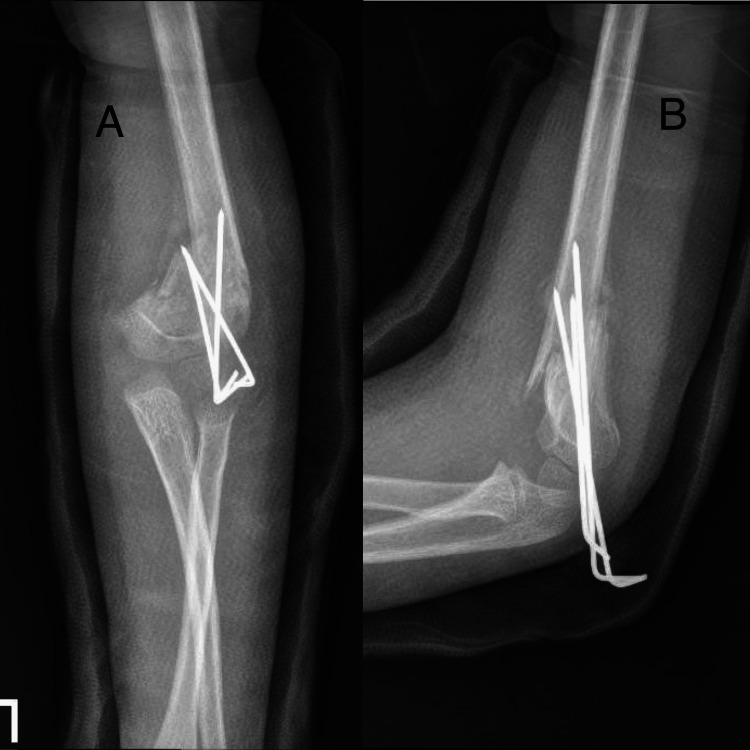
X-ray immediately before revision surgery (day 15 post initial CRPP) (A) anteroposterior view; (B) lateral view CRPP: closed reduction percutaneous pinning
